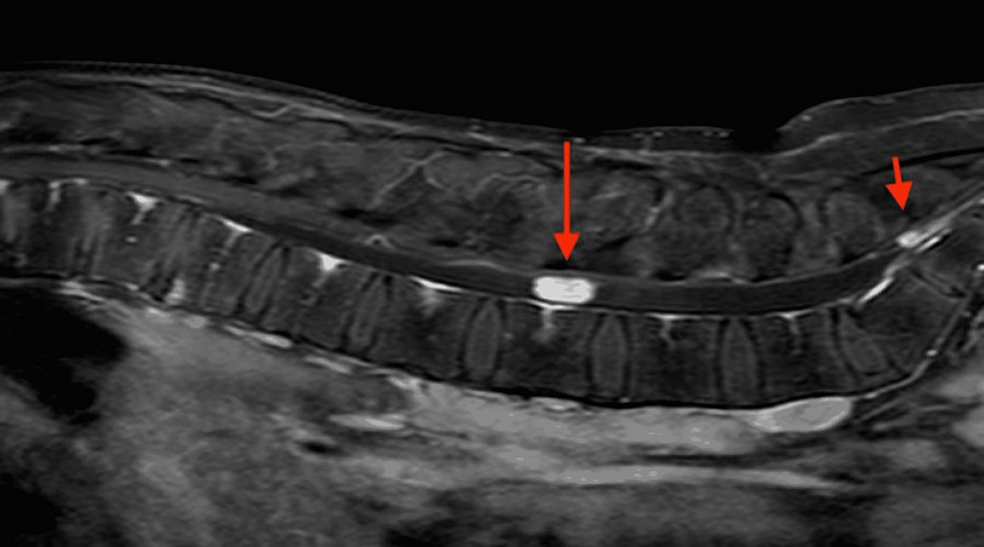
Sagittal T1 Gd+ MRI of the lumbar spine Sagittal T1 Gd+ MRI of the lumbar spine showing two intradural cauda equina tumours, a larger one at the L3 level (big arrow) and a smaller one at the S2 level (small arrow).
